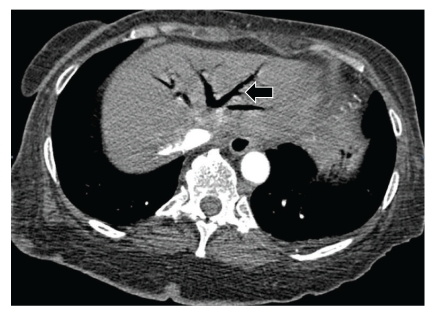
CT image of the liver shows air delineating the hepatic venous system (*arrow*).
